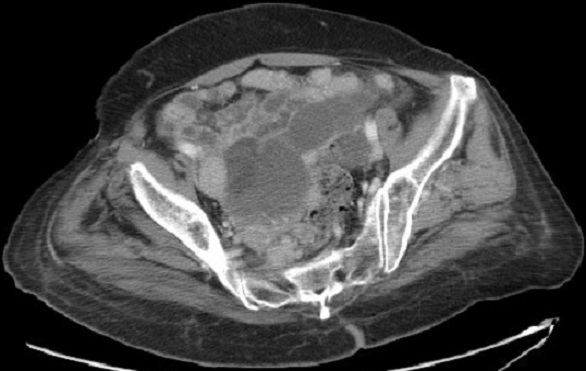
CT scan of the pelvis showing after 3 cycles of chemotherapy a stability according to RECIST 1.1
